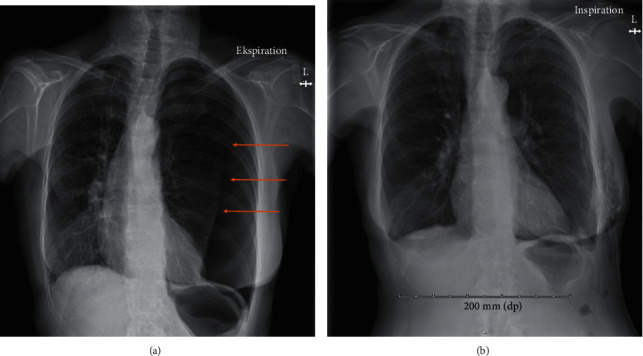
(a) Chest X-ray showing tension pneumothorax of the left lung. The first chest X-ray showed a large left-sided rim pneumothorax, extending along the entire lateral border of the lung, from the base to the apex, and with a maximal width of 6 cm at the base of the left lung. (b) Chest X-ray showing subcutaneous emphysema. A postintervention chest X-ray showed an intercostal drain placed at about the 8th intercostal space on the left side. A near complete regression of the pneumothorax is seen, with the lung parenchyma again extending to the borders the left hemithorax and a smaller residual rim apicolaterally measuring 12 mm. In the soft tissues at the puncture point, multiple diffuse hypodense areas are seen, resembling subcutaneous emphysema.
